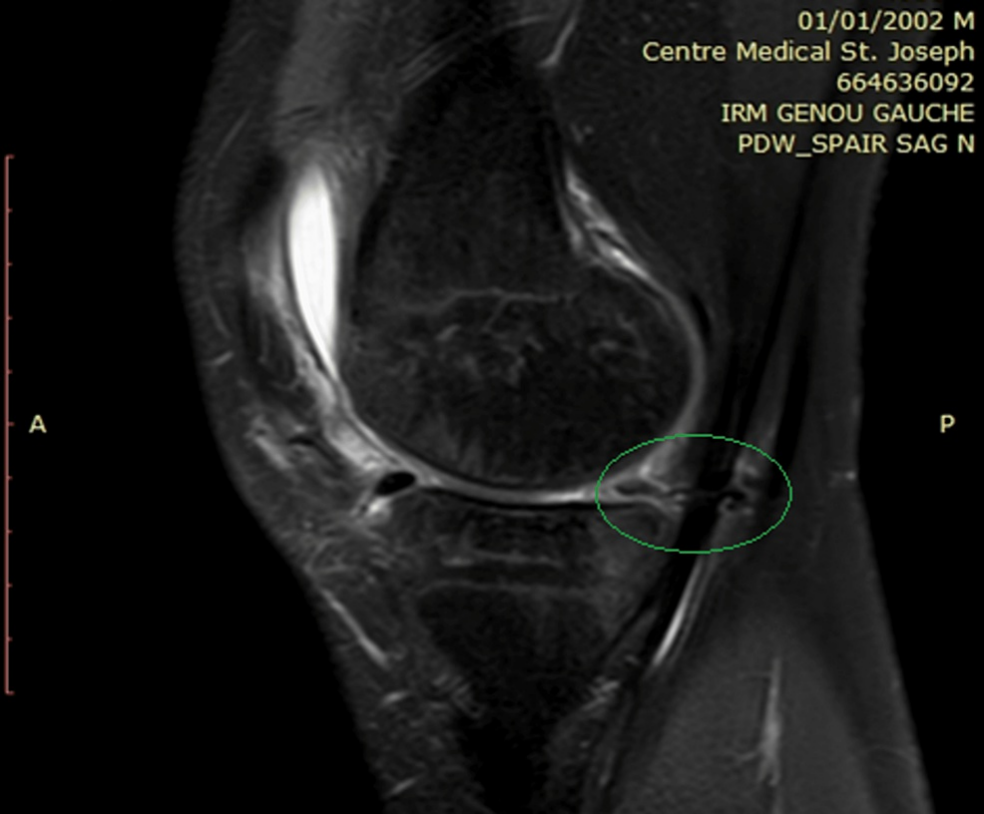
MRI of the left knee showing entrapment of semimembranosus tendon by medial meniscal suture
